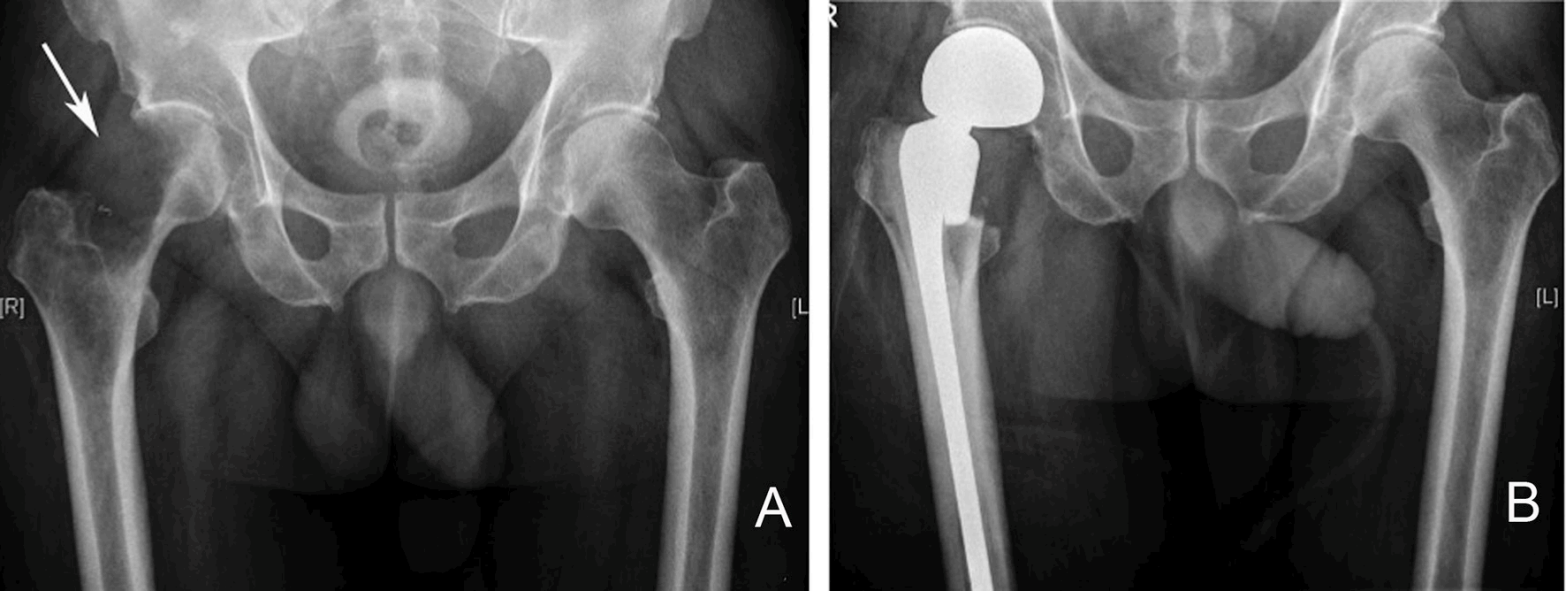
Right proximal femur metastatic melanoma treated with a calcar replacing hemiarthroplasty. (A) Large destructive lytic metastatic lesion involving the head and neck of the right femur. (B) X-ray post reconstruction with a calcar replacing hemiarthroplasty implant.
